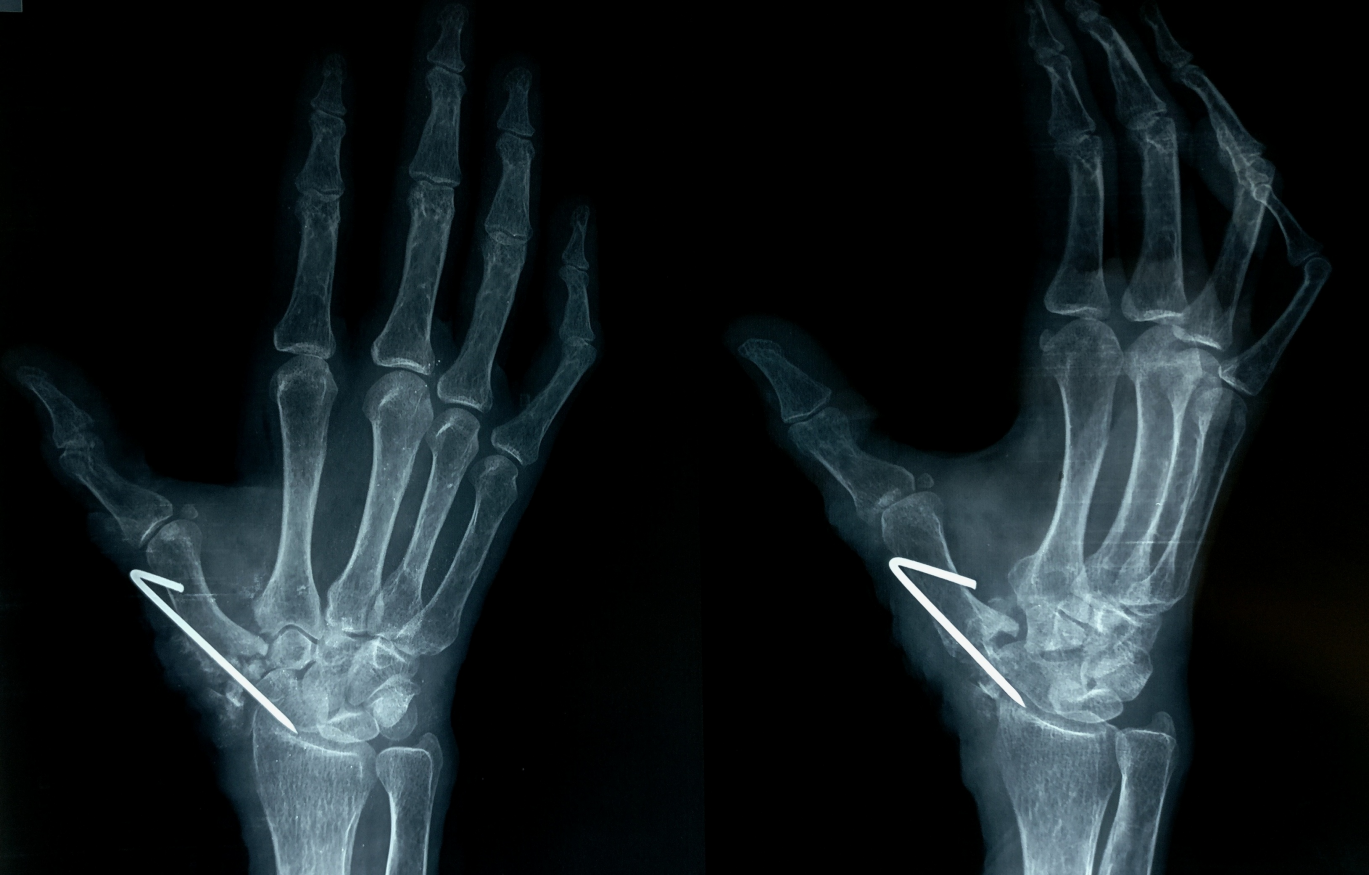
Postoperative radiographs (antero-posterior and oblique views) Image showing K-wire in situ and cavity filled up with trapezium graft.
